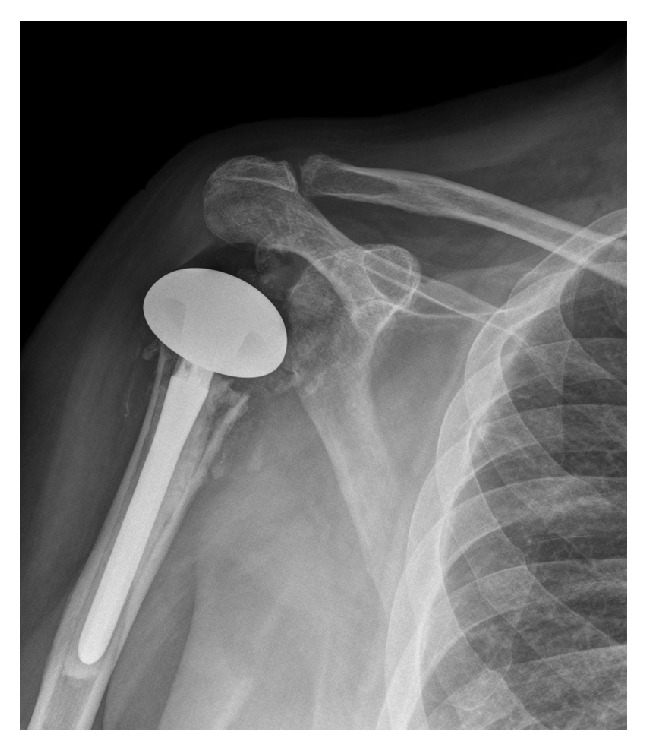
Radiograph showing the hemiarthroplasty.
